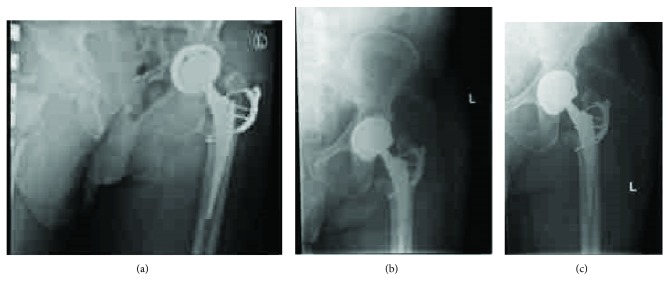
Pelvic X-ray of the left hip one postoperative day of THA revision demonstrating satisfactory positioning of the implants and placement of trochanteric cable plate. Wire was placed around the inferior portion of the Zimmer 51 mm short stature plate at the lesser trochanter and below. A second wire was placed at the upper trochanter at the implant junction. A third wire was placed around the greater trochanter.
